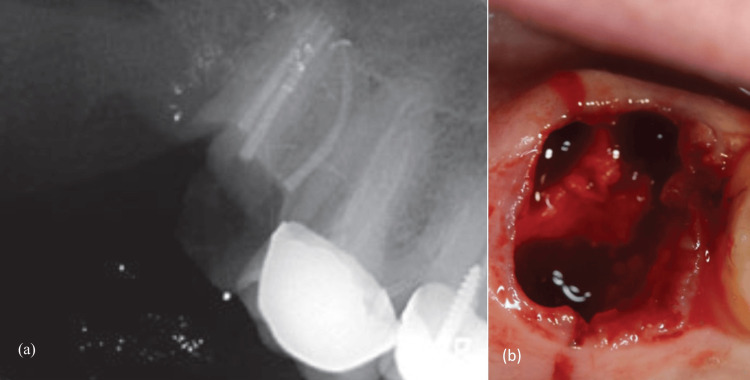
Clinical case example: (a) Pre-treatment radiograph; (b) atraumatic tooth extraction.
